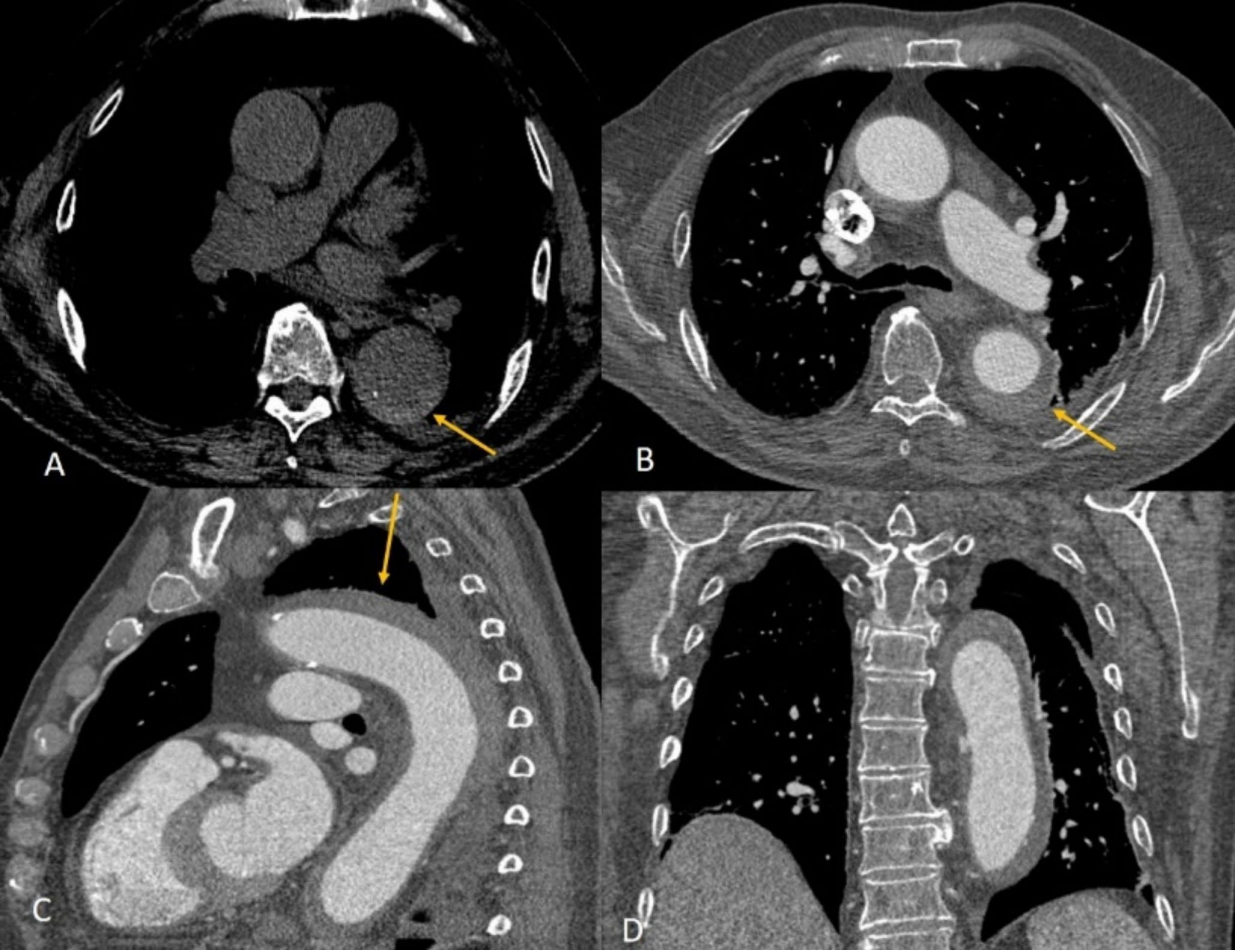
Intramural hematoma. Non-enhanced axial CT image depicts a near-circumferential high attenuation soft tissue area (yellow arrow) within the wall of a dilated descending aorta. The blood in the lumen of the aorta is lower in attenuation compared to the aortic wall on this non-contrast image. There are also intimal calcifications as this type of AAS is considered an atypical form of aortic dissection due to hemorrhage into the aortic wall from leaking vasa vasorum without an intimal tear (A). Axial contrast enhanced CT angiography image shows opacification of the aortic lumen with persistence of the abnormal circumferential soft tissue within the aortic wall (yellow arrow) (B). Sagittal (C) and coronal (D) CT angiography images demonstrate the extent of the intramural hematoma
